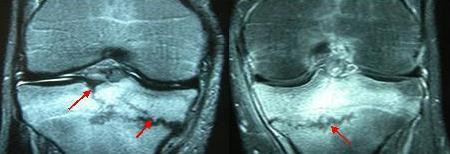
Coronal MR images show a fracture line at the medial side of left tibia which extends into the lateral half. Fracture at the right side extends into the articular surface.
